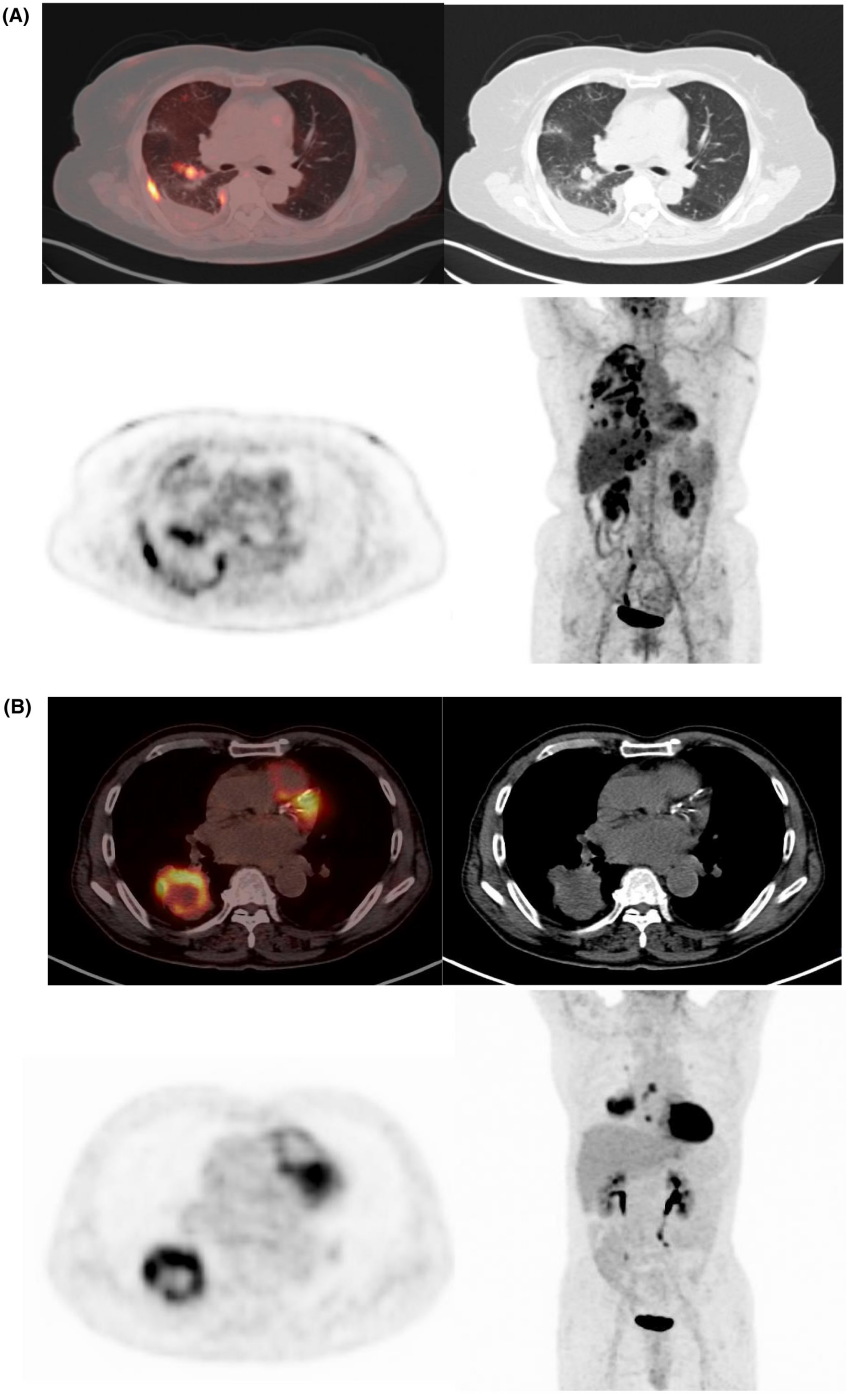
(A) Adenocarcinoma, Stage IVA, SUV_max_ was 5.46, SUL was 4.35, MTV was 7.77 cm^3^. A low MTV and TLG value of the primary site with pleural and mediastinal lymph node metastases occurred. The number of total CTCs was 20/5 mL, Epithelial CTCs was 9/5 mL, mixed CTCs was 10/5 mL and mesenchymal CTCs was 1/5 mL. (B) Squamous carcinoma, Stage IIIB, SUV_max_ was 11.32, SUL was 9.8, MTV was 50.54 cm^3^, TLG was 240.17 cm^3^, and central necrosis of the primary lesion resulted in reduced MTV and TLG. The number of total CTCs was 6/5 mL, Epithelial CTCs was 6/5 mL, mixed CTCs and mesenchymal CTCs was 0/5 mL.
